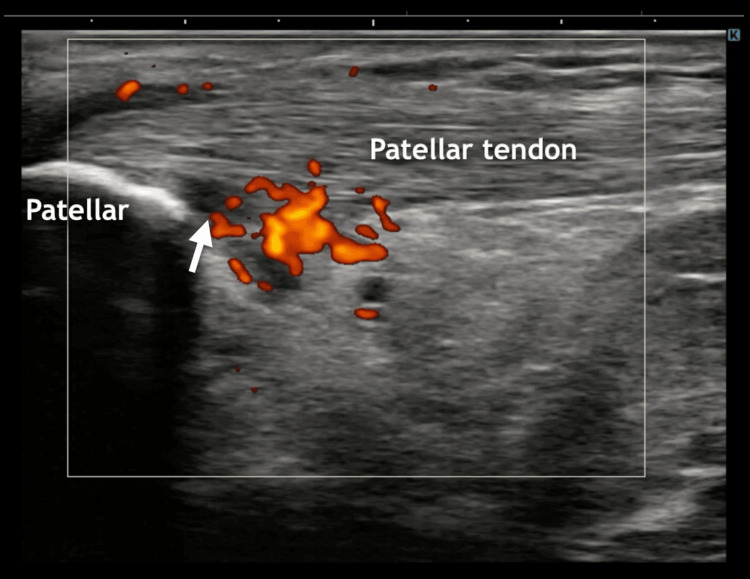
Long-axis ultrasound image of the left patellar tendon A slightly hypoechoic area with increased vascularity (arrow) is observed in the deep layer of the proximal portion of the patellar tendon. Despite these findings, no tenderness was observed at the site. The main body of the tendon maintained a normal fibrillar echotexture, suggesting preserved structural integrity.
